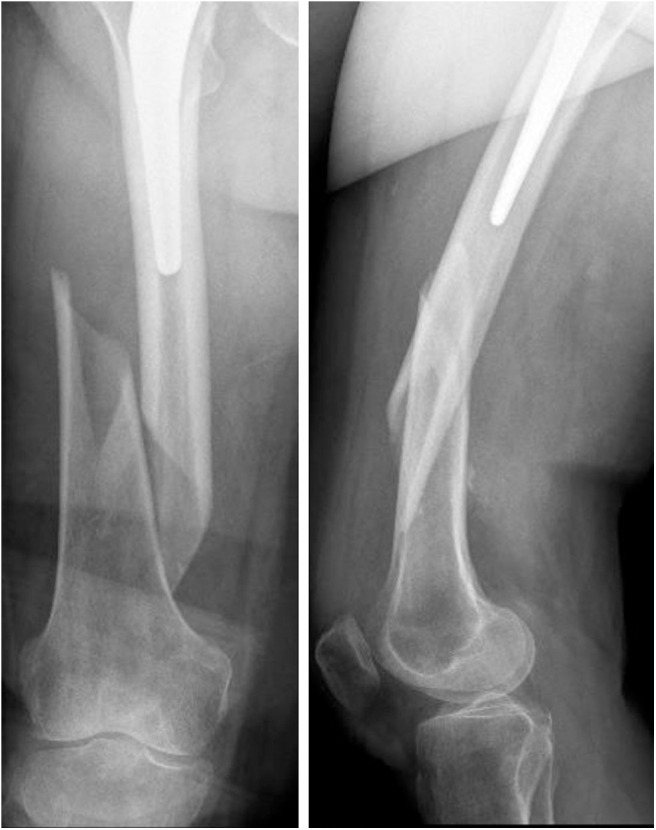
Anteroposterior (Left) and lateral (Right) x-ray images of the periprosthetic fracture.
